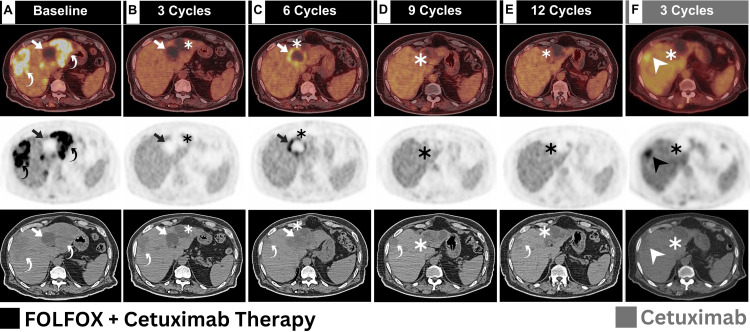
Serial hepatic transaxial images (composed of fused PET/CT, axial PET, and axial CT images for each panel) captured from baseline and following each of the three cycles of therapy received Serial fused [^18^F]FDG PET/CT, PET, and CT liver images were captured from baseline and following each of the three cycles of therapy received. A: At first, several hepatic lesions of various density and metabolic activity were observed (annotations). B: Following the third cycle, all previous hypermetabolic lesions were resolved, yet another hypometabolic lesion (asterisk) emerged near the prominent segment IV necrotic lesion (arrow). C: following the sixth cycle of therapy, the prominent segment IV lesion has established a hypermetabolic rim (arrows), while the segment II lesion has diminished (asterisk). D: After the ninth cycle, morphologic regression of the segment II lesion was witnessed (asterisk), along with a complete response of the aforementioned segment IV lesion. E, F: Additional therapy courses resulted in gradual progression of segment II lesion (asterisks), along with interval establishment of hypermetabolic process in the previously persistent segment VIII hypodensity indicative of viable metastasis (arrowhead). FOLFOX - folinic acid, fluorouracil, oxaliplatin; [^18^F]FDG PET/CT - [^18^F] fluorodeoxyglucose positron emission tomography/computed tomography
